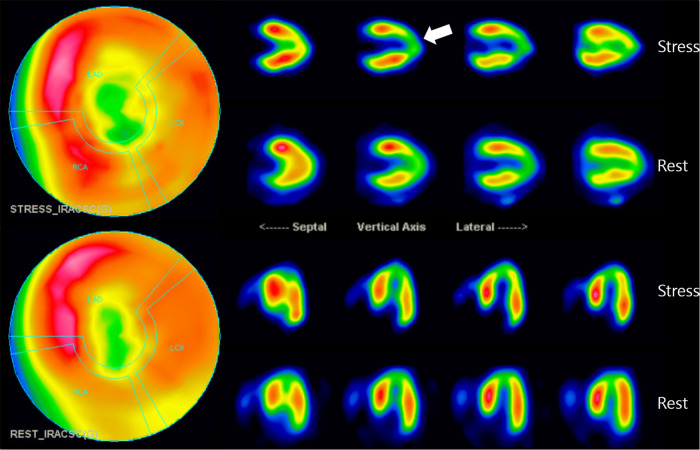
Myocardial perfusion SPECT imaging: SPECT scan showed medium-sized, non-transmural infarction around the apex (whitish arrow). This defect correlates to the downstream myocardium to the bridging segment of the mid-LAD, as marked by arrows. LAD, left anterior descending coronary artery; SPECT, single photon emission computed tomography.
